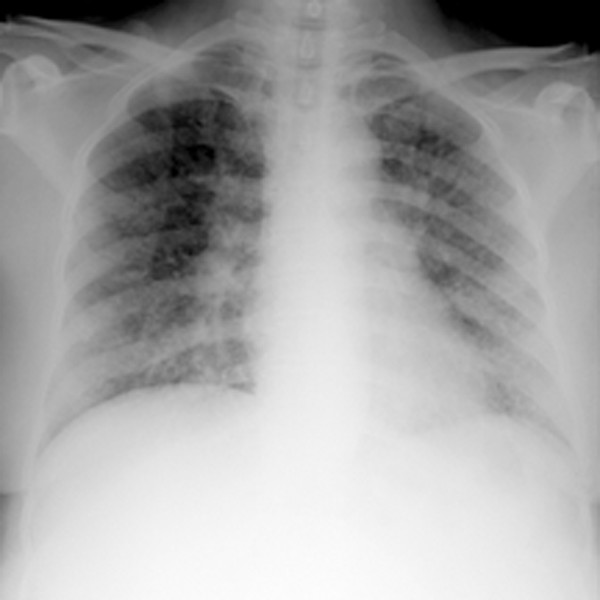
Chest radiograph showing diffuse nodular and patchy pulmonary infiltrates, and right hilar widening.
